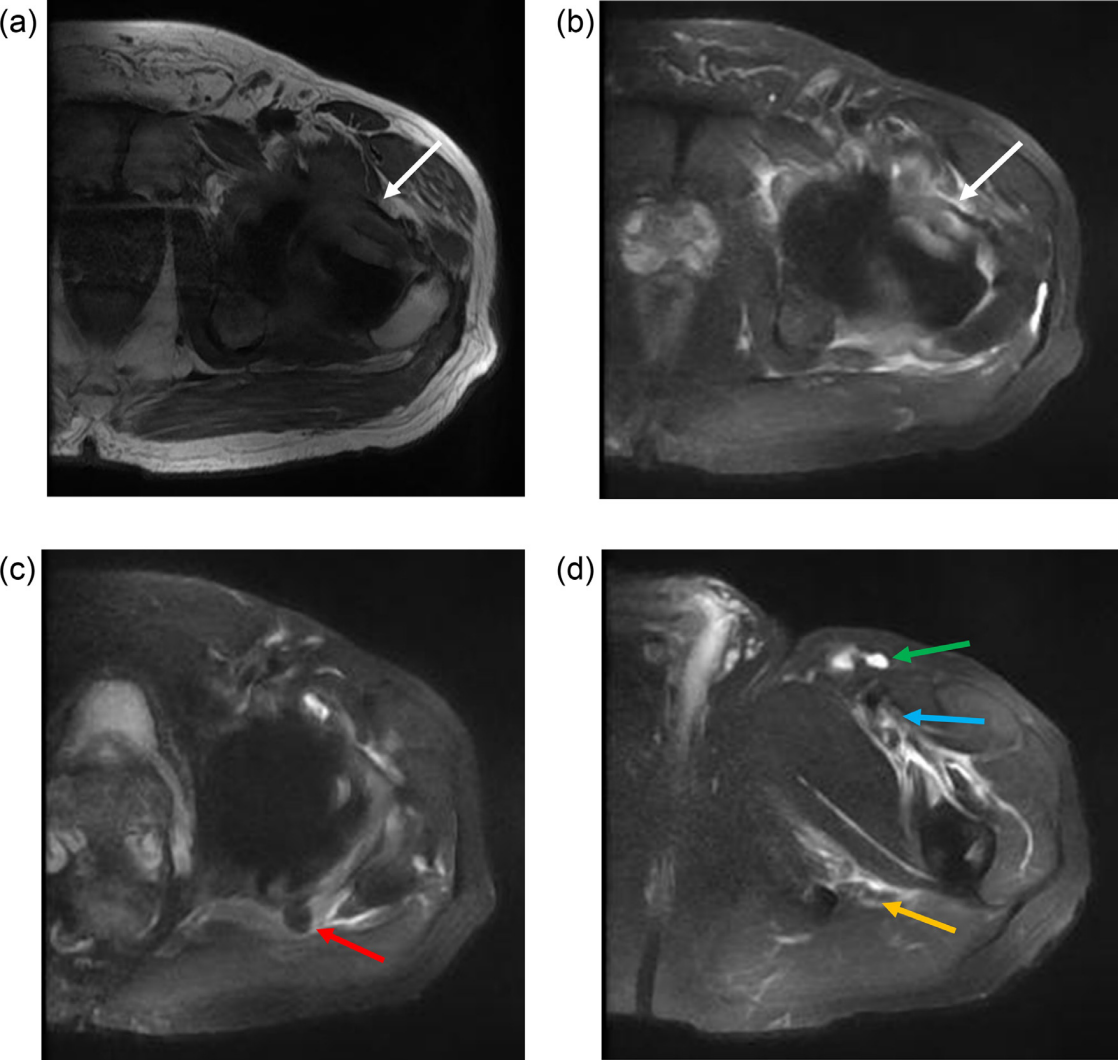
(a) Axial T1 and (b) axial STIR images from MRI of the left hip using a MAVRIC protocol for metal artifact reduction. These demonstrate distention of the hip joint capsule due to complex fluid/synovitis, best seen along the femoral neck anteriorly (white arrows). There is surrounding edema. (c) Axial STIR image shows a metallic particle posterior to the left hip (red arrow). (d) Axial STIR image shows edema-like signal around the left proximal femur extending to the femoral vessels (blue arrow) and sciatic nerve (orange arrow). Non-enlarged hyperintense left inguinal lymph nodes are noted (green arrow).
